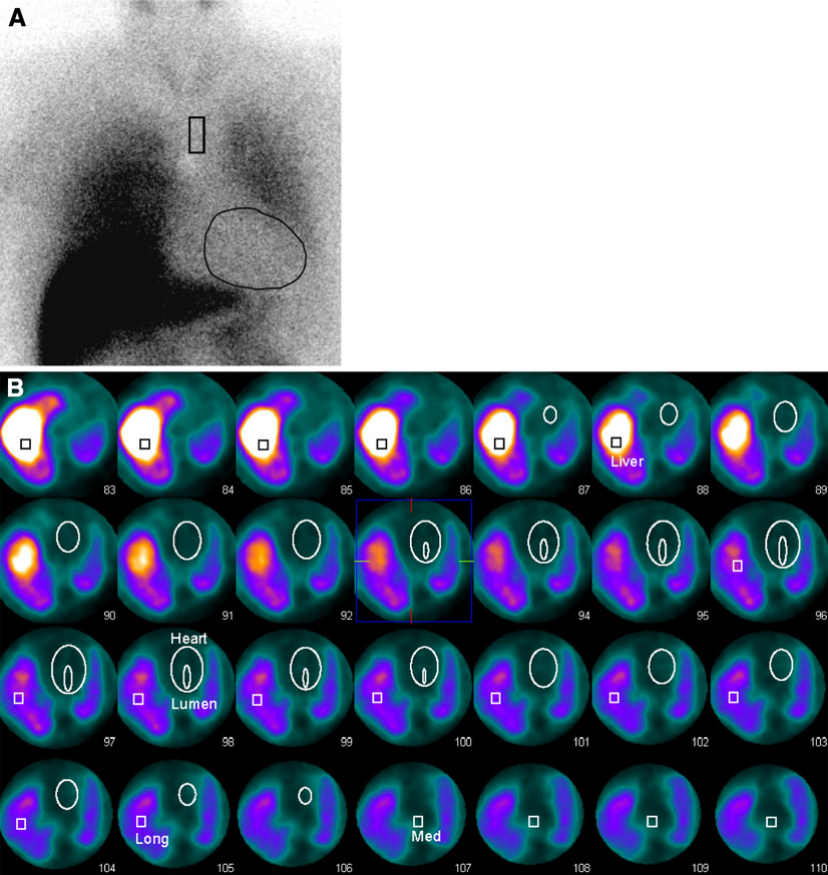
I-123 MIBG acquisition of a patient from the NDD population (age 60 years). This patient suffers from Parkinson’s disease and diabetes mellitus, but has no history of cardiac diseases. A The early planar image shows a severely reduced accumulation of I-123 MIBG in the cardiac region, but intense uptake in the liver and lung. (eHM = 1.21, dHM = 1.15, WOR = 0.42). B The VOIs for the left ventricle, lumen, mediastinum, right lung and liver are drawn into the SPECT acquisition. Based on these VOIs indices for the HM (eHM = 1.09, dHM = 0.91, WOR = 3.75), MM (eMM = 0.41, dMM = 0.26, WOR = −0.31) and ML (eML = 0.60, dML = 0.52, WOR = 0.08) are determined
